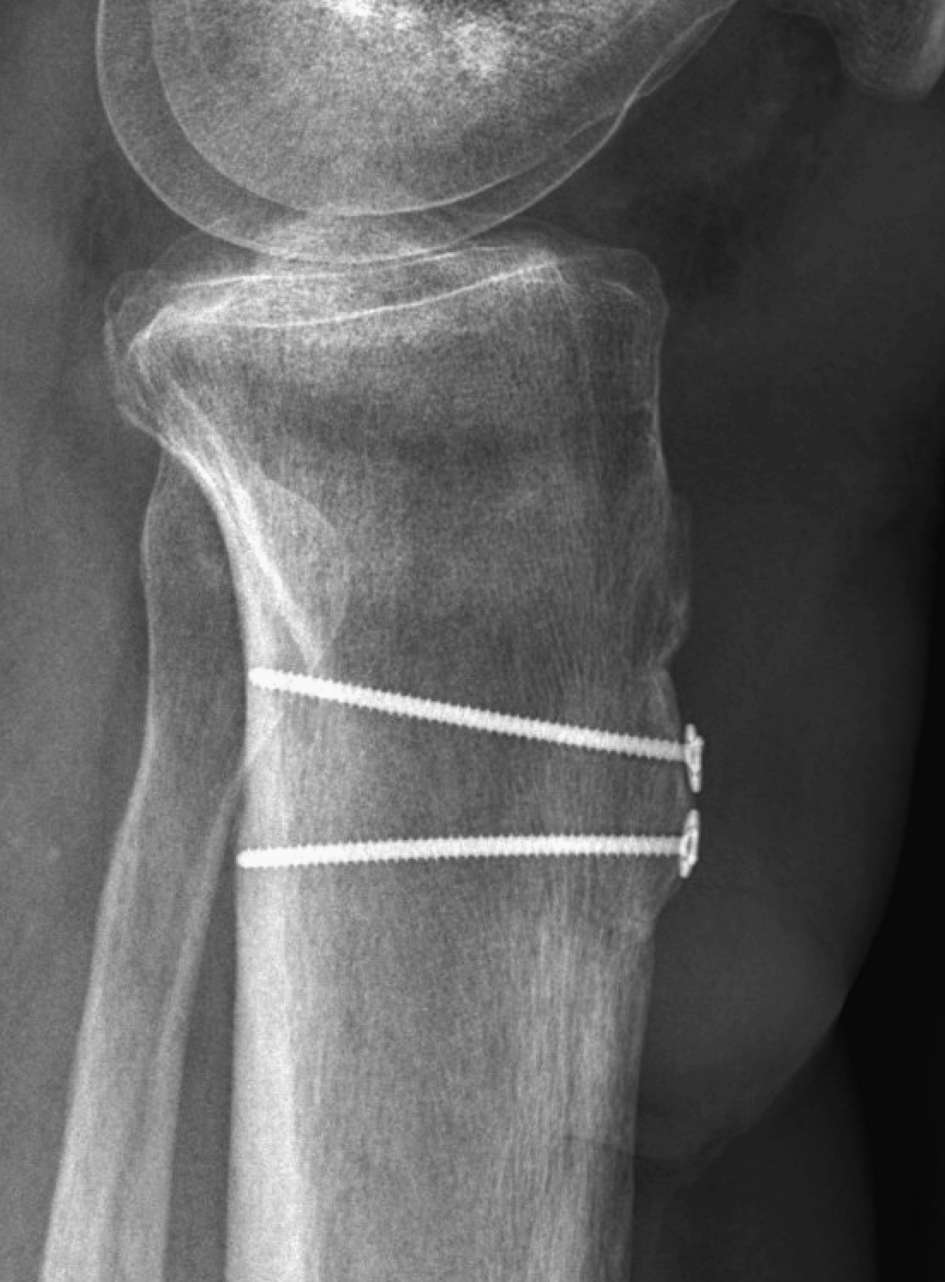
Eight-month lateral radiograph of the left tibia demonstrating bony incorporation of calcaneus bone block allograft
